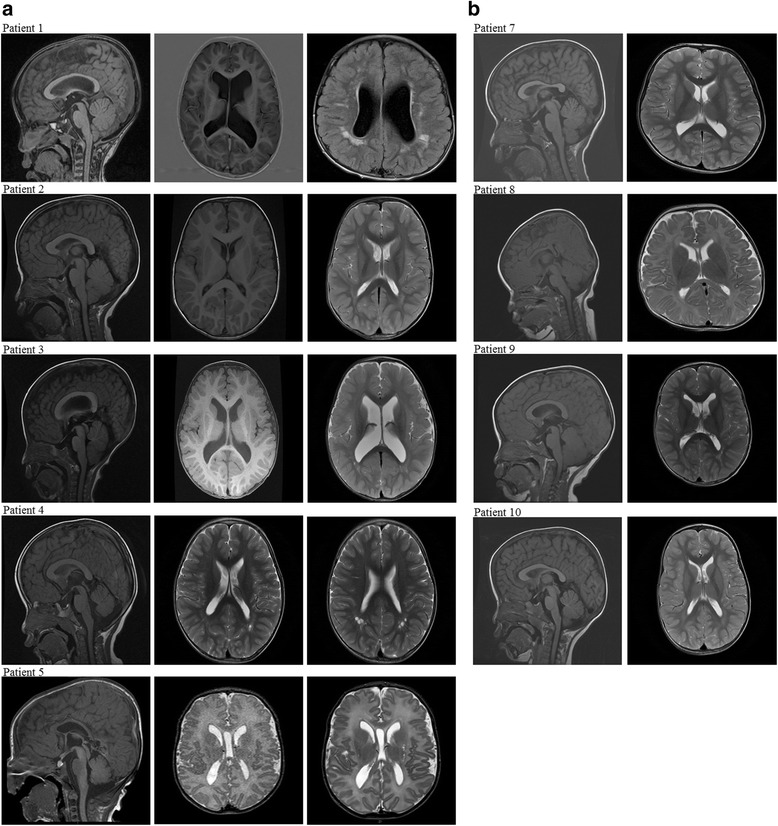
MRI of patients with mutations in the PI3K-AKT-mTOR pathway. a MRI in patients 1–5 showing megalencephaly, polymicrogyria and periventricular white matter signal abnormalities. Ventriculomegaly was observed in patients 1, 3 and 5. b MRI in patients 7–10 showing megalencephaly, without other abnormalities. MRI was not available for patient 6 because the family declined the MRI
